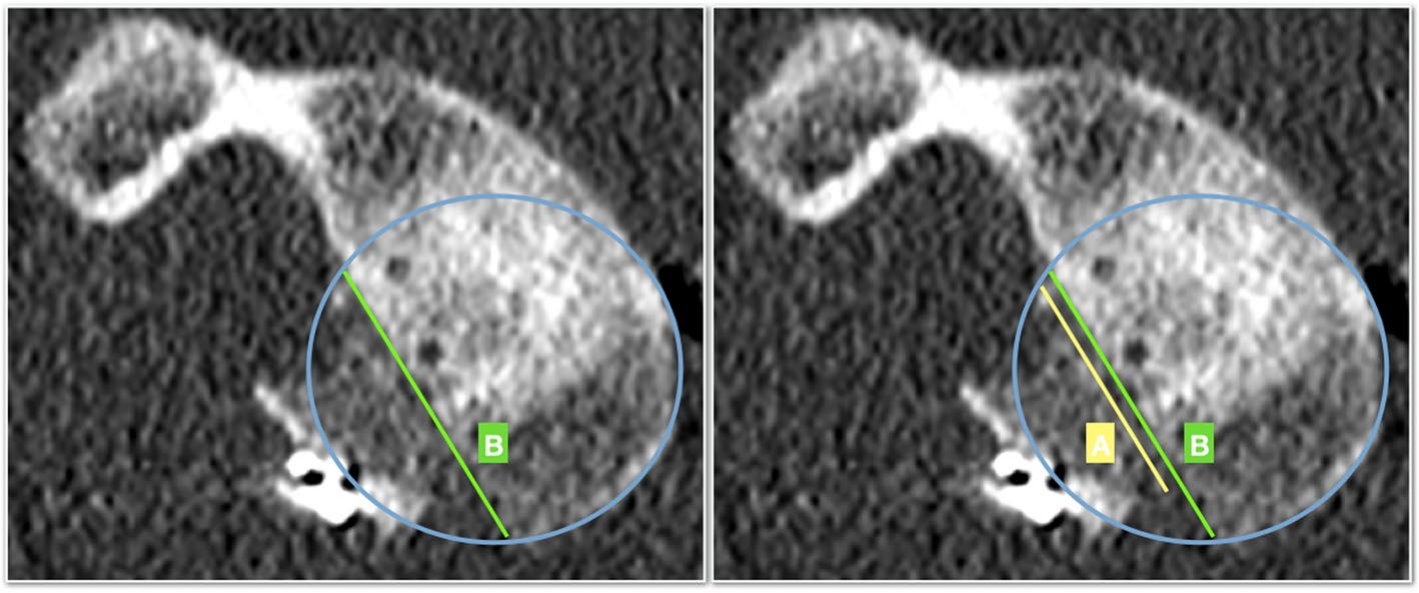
a postoperative CT en-face image of the left shoulder of a subject with anterior shoulder instability following an arthroscopic bone block procedure. The bone-block position is evaluated using the “Defect coverage index method”. First the length of the bone defect is measured (green line, B) (A). Secondly, the amount of graft covering the glenoid defect is quantified
